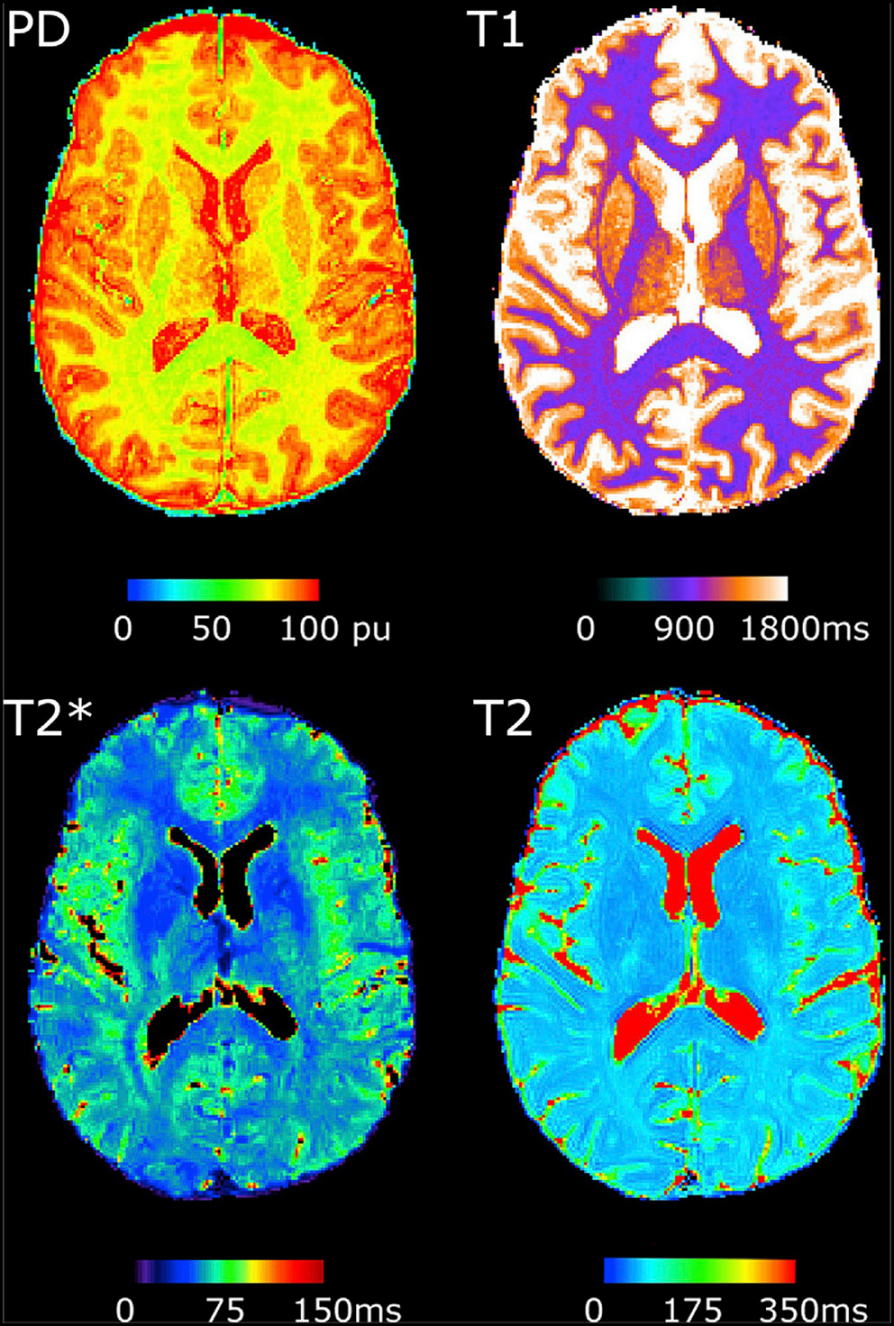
Examples of quantitative MRI maps of a single subject. PD, Proton density. The figure has been published under a Creative Commons license (https://creativecommons.org/licenses/by-nc-nd/4.0/) by Gracien et al. (3).
